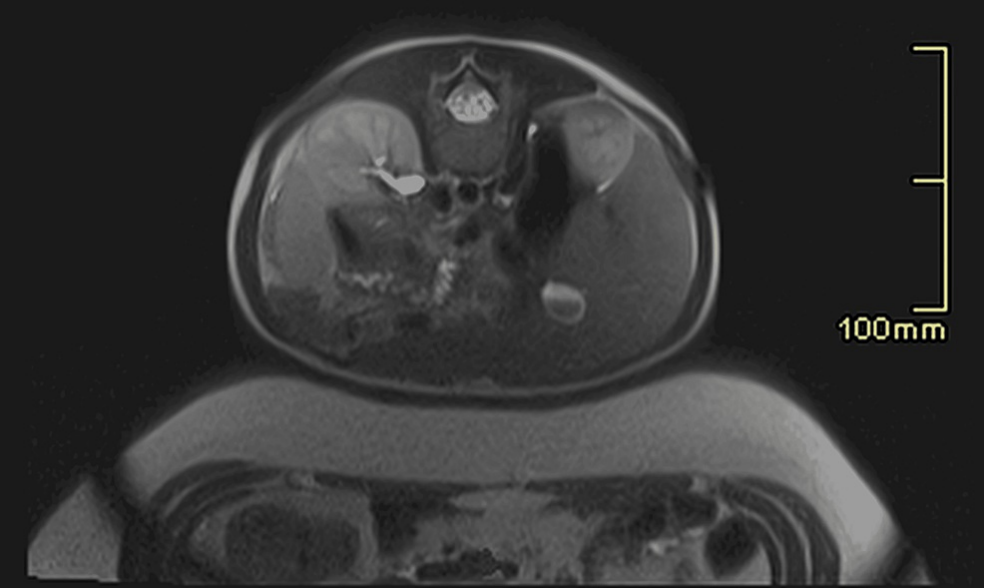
1.5 T MRI T2-weighted axial imaging of the same child’s abdomen demonstrating that images of diagnostic quality are achievable using our abdomen-to-abdomen method.
